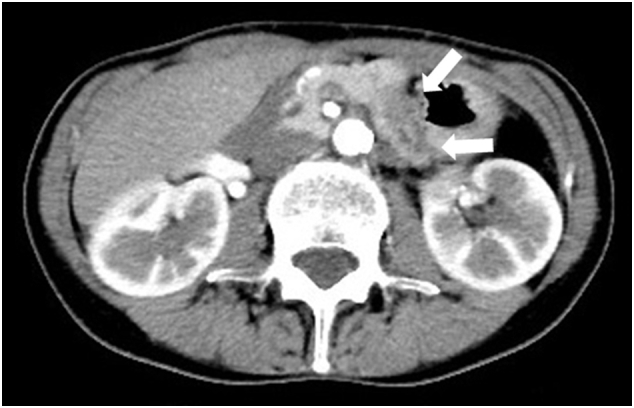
Abdominal dynamic CT scan findings. A: A low-density mass is observed (white arrow) during the arterial phase of the study. CT: computed tomography.
